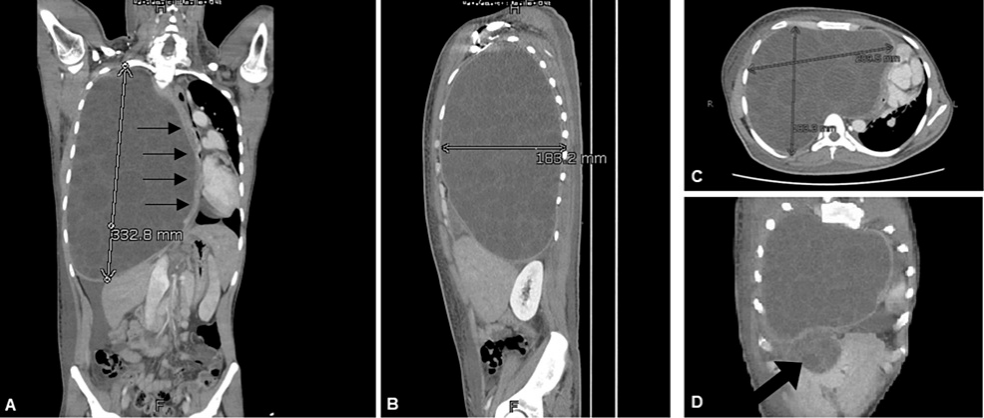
CT chest coronal sections (A and D), sagittal section (B), and axial sections (C) CT chest shows a huge hydatid cyst containing innumerable daughter cysts occupying the entire right hemithorax and crossing the midline with a significant cardio-mediastinal shift towards the left (thin black arrows in A) and significantly attenuated superior vena cava. The total size of the cysts measures 183 X 209.5 X 333 mm in maximum anteroposterior, transverse, and craniocaudal dimensions as shown in the images. (D) shows the exophytic cyst abutting the liver (thick black arrow).
